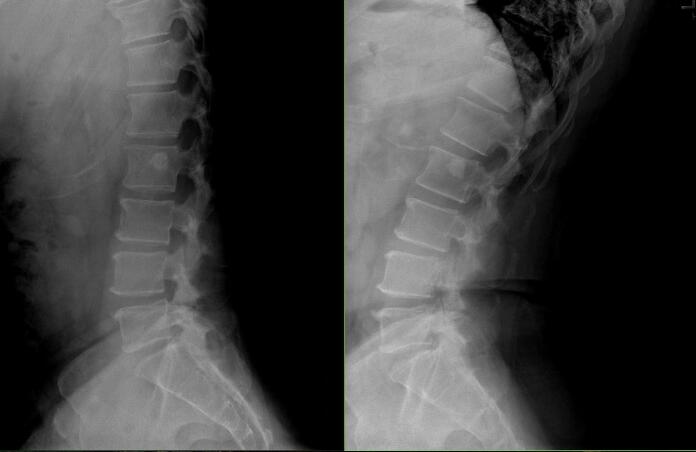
Flexion and extension lateral lumbar radiographs showing no change in alignment or instability.
